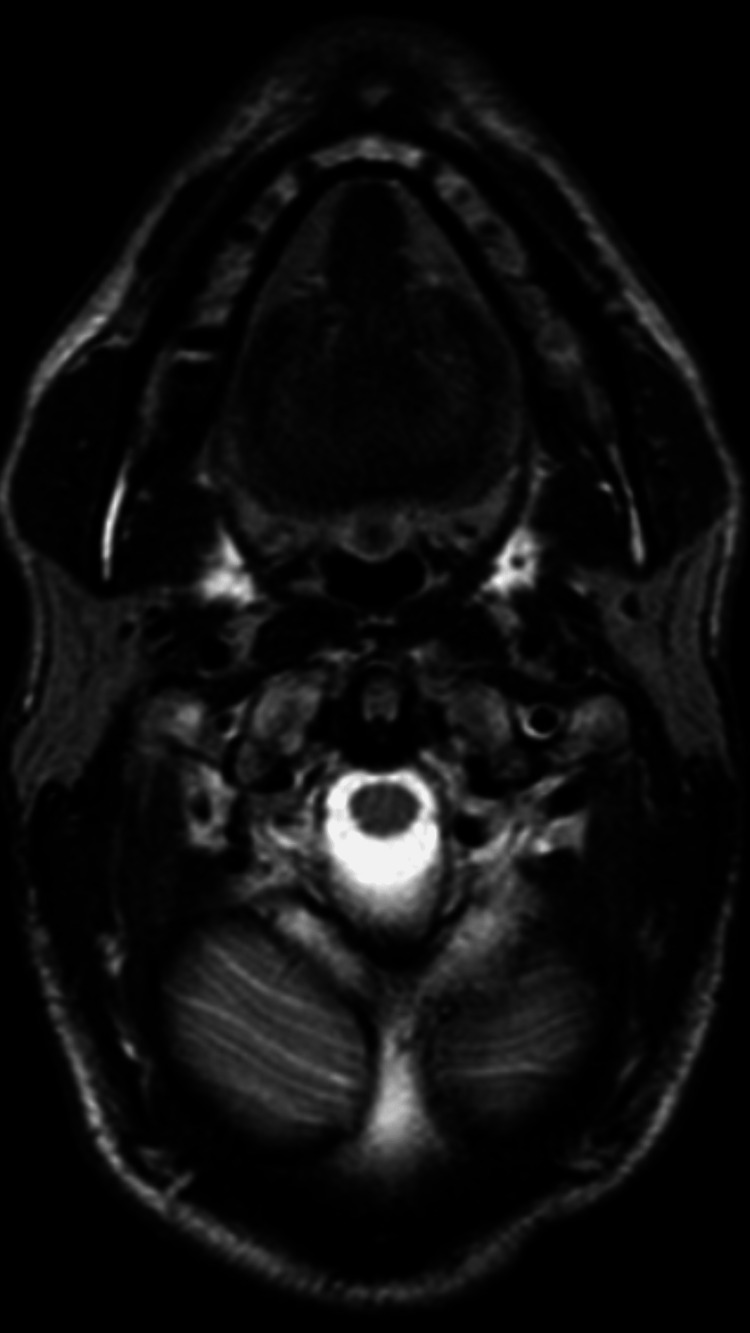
Axial MRI T2w showing no abnormalities arising from the left parotid gland or parapharyngeal space (PPS)
